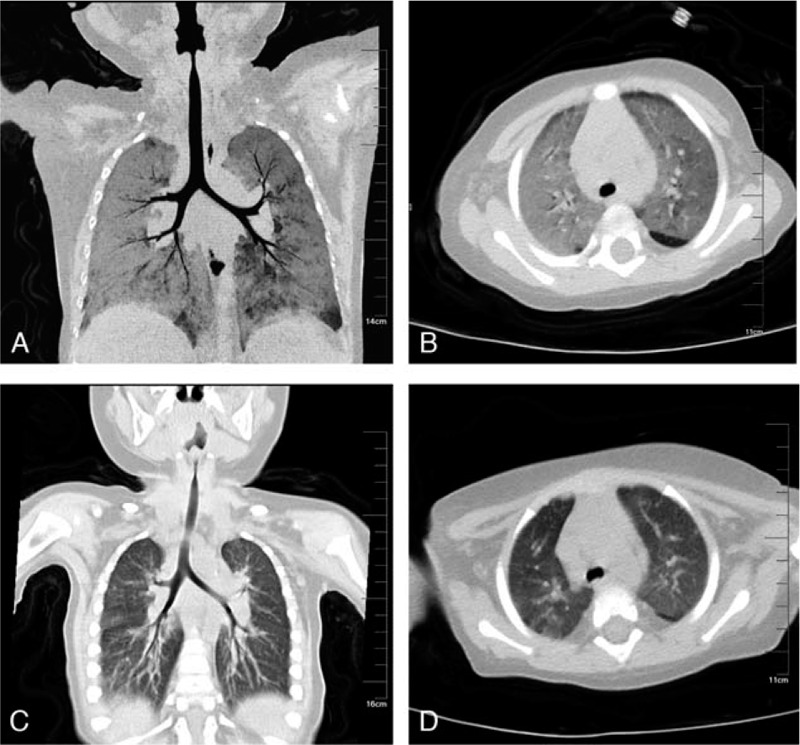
Chest CT before (A/B) and after (C/D) treatment. CT = computed tomography.
